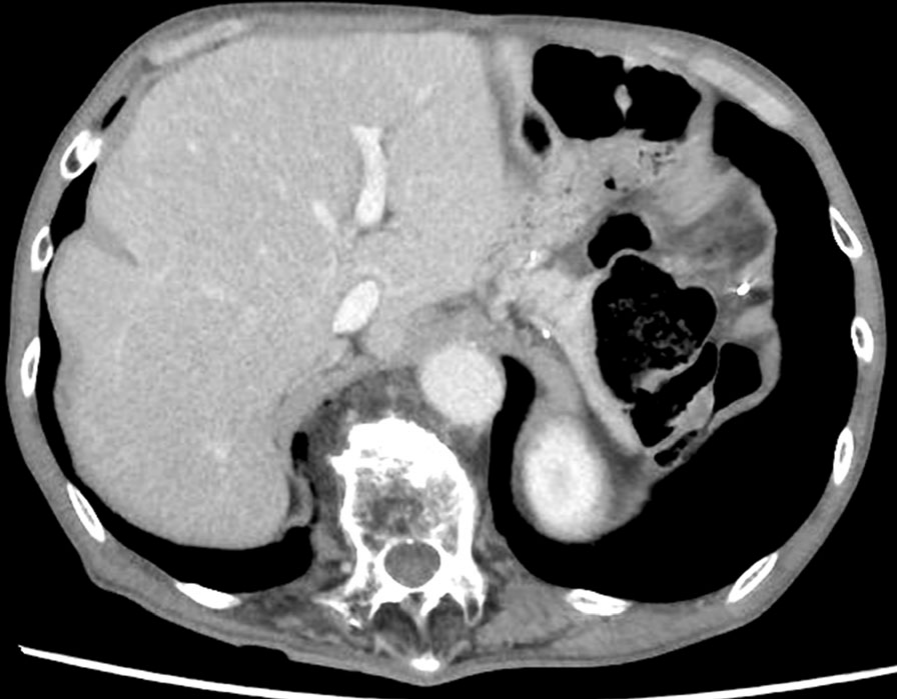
Abdominal computed tomography (CT) findings after 12 cycles of nivolumab. No liver mass lesions are seen on abdominal enhanced-contrast CT
